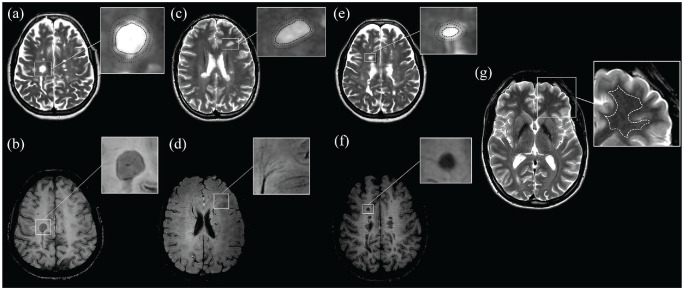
Region-of-interest (ROI) (dashed ROI: relaxometric analysis of the lesion core; dotted ROI: relaxometric analysis of the periplaque area (PPA)) placements are demonstrated on synthetically generated T2-weighted contrasts (repetition time (TR)/echo time (TE): 4500/100 ms) (a, c, and e). The corresponding susceptibility-weighted imaging (SWI) data were used to discriminate paramagnetic rim lesions (PRLs), SWI-isointense lesions (SILs), and lesions with diffuse SWI-hypointense signal (DSHLs) (b, d, and f). A PRL (a and b) with respective ROIs (a) is shown in a 24-year-old male patient with relapsing multiple sclerosis and 0.4 years of disease duration with an EDSS of 0 and an MSSS of 0.67. An SIL (c and d), DSHL (e and f), and the normal-appearing white matter (NAWM) (g) with respective ROIs (c, e, and g) are shown in a 42-year-old female patient with secondary progressive multiple sclerosis and 16.9 years of disease duration with an EDSS of 4.0 and an MSSS of 3.65.
